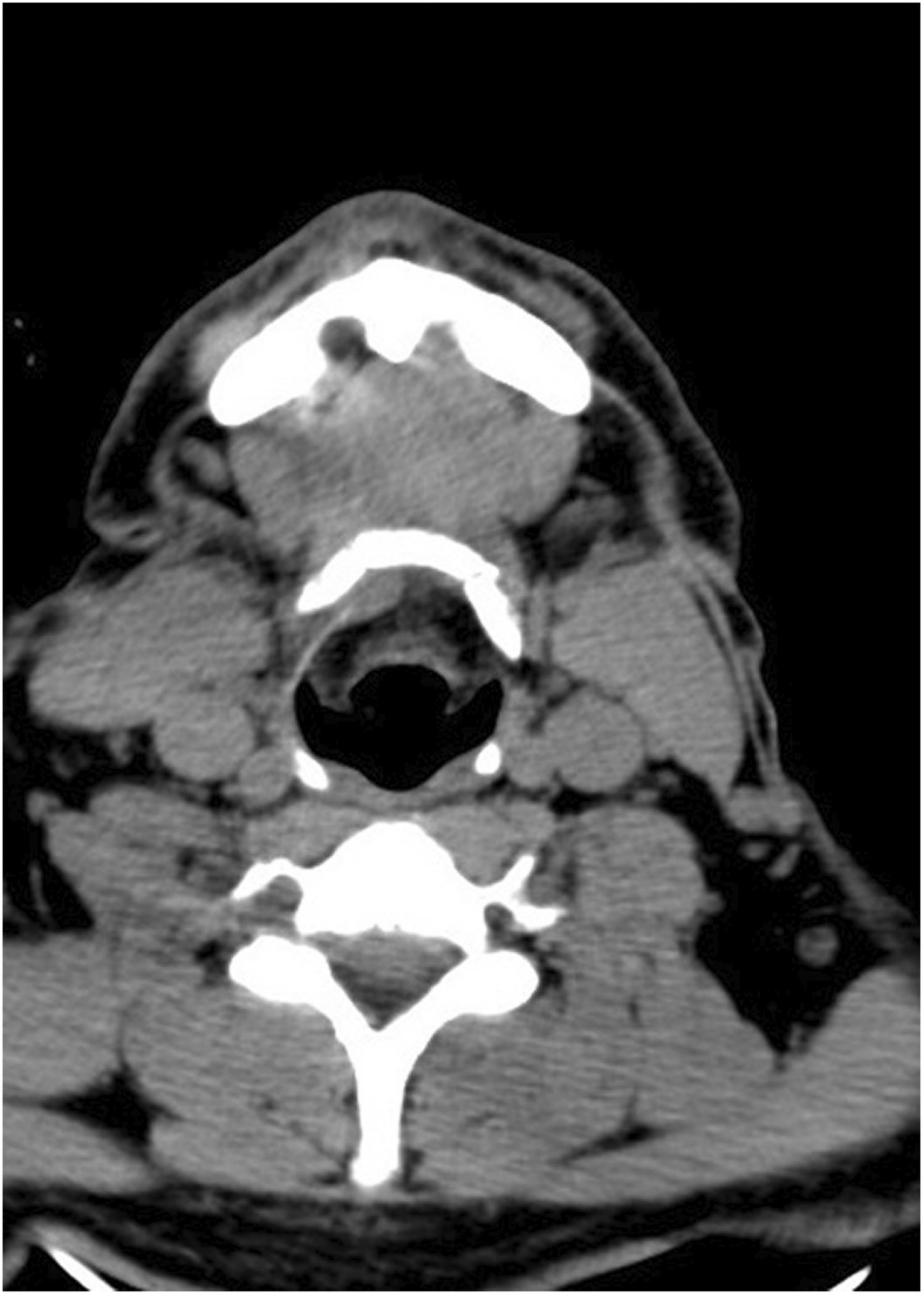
The axial view of neck CT scan shows no space‐occupying lesions or any other pathologic finding.
